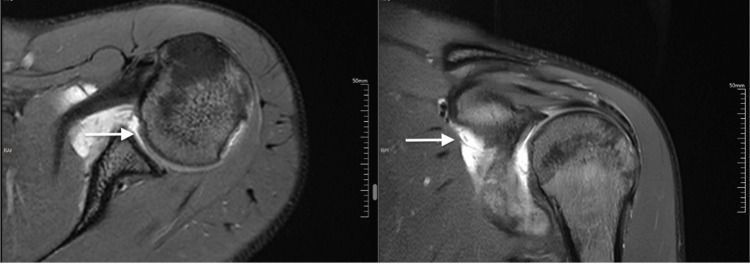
MRI (axial and coronal) section shows labrum tear with minimal joint effusion and subscapularis tendon tear, respectively (proton density/short TI inversion recovery sequences, white arrows).
